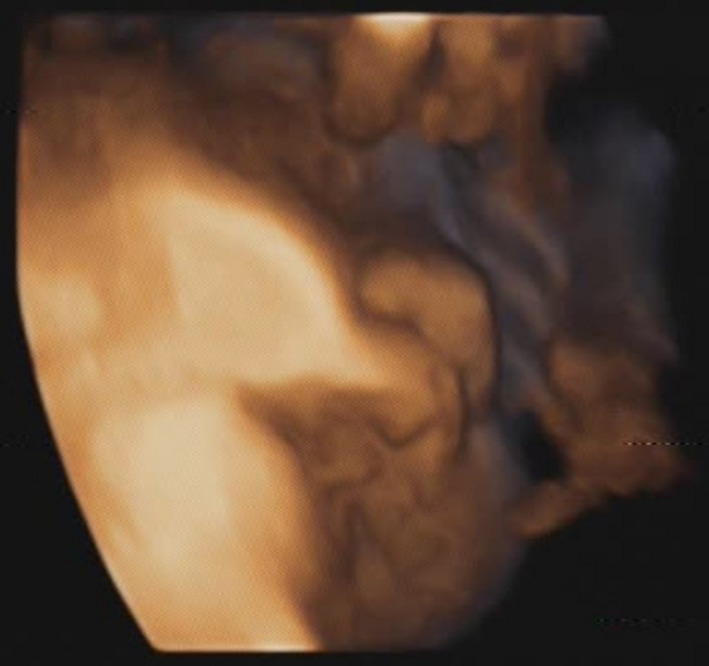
Prenatal fetal three‐dimensional ultrasound imaging at 35 + 2 wk of gestation further demonstrating the degree of micrognathia and retrognathia present
